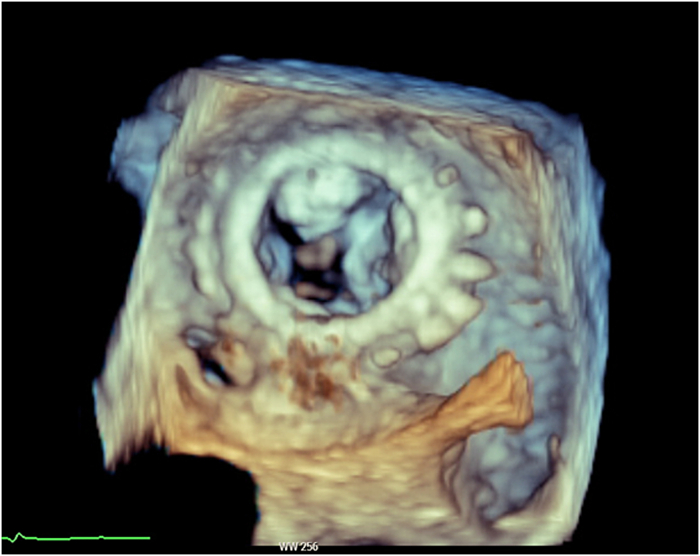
Three-Dimensional View of the Stenotic Mitral Valve Prosthesis Three-dimensional transesophageal echocardiography of severely degenerated bioprosthetic mitral valve showing thickened, sclerotic leaflets with restricted opening.
